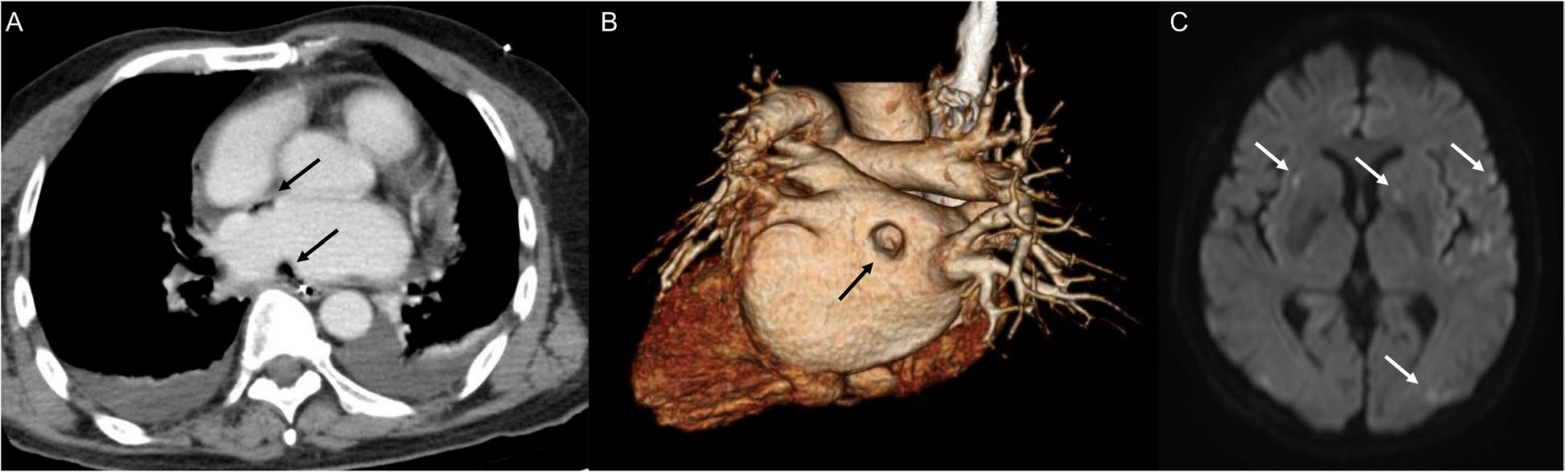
Preoperative enhanced computed tomography showed the presence of air in the left atrium (A). 3D image showed contrast defect in the posterior wall of the left atrium (B). Magnetic resonance imaging showed multiple cerebral infarction (C)
